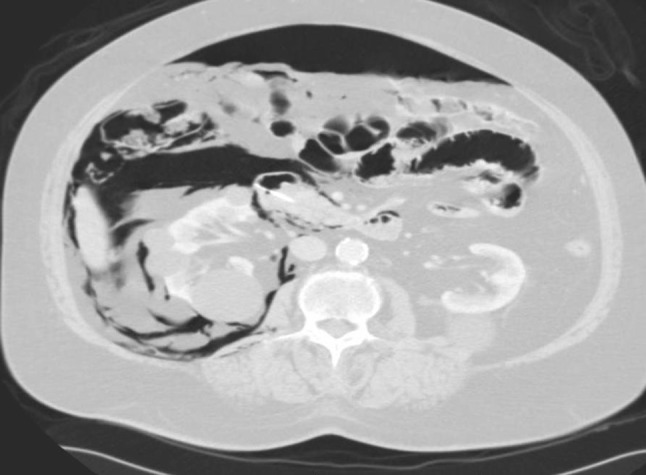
Case 1* Abdominal CT-scan showing intra- and retroperitoneal free air
